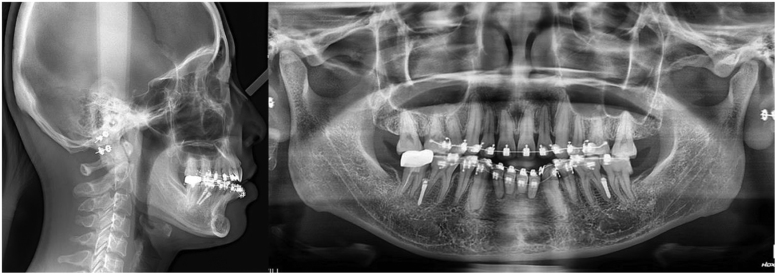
Panoramic radiograph at presentation.
